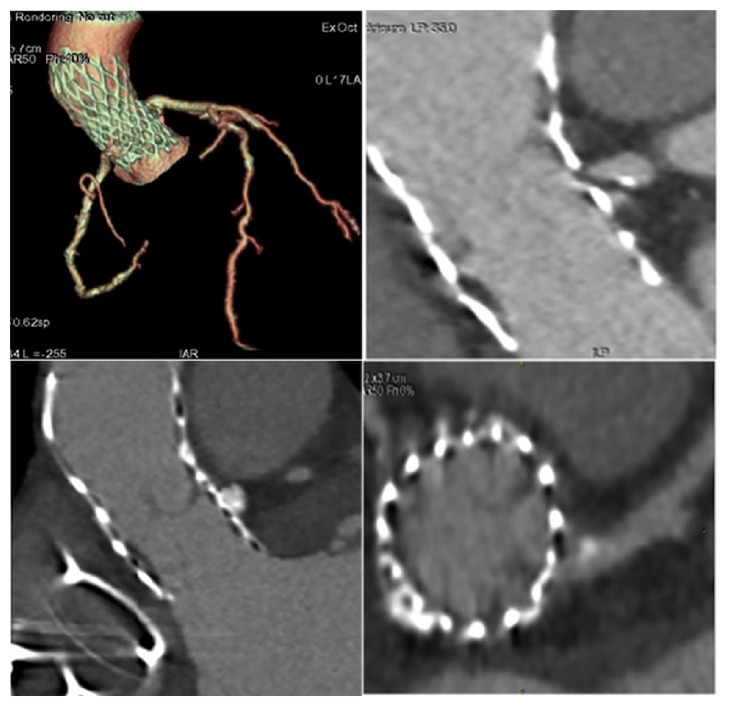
Follow-up gate CT scan demonstrating bioprosthesis migration with respect to left coronary. (S1-S2). Figure 5 S2 upper right: evident presence of the stenosis of the left coronary ostium.
